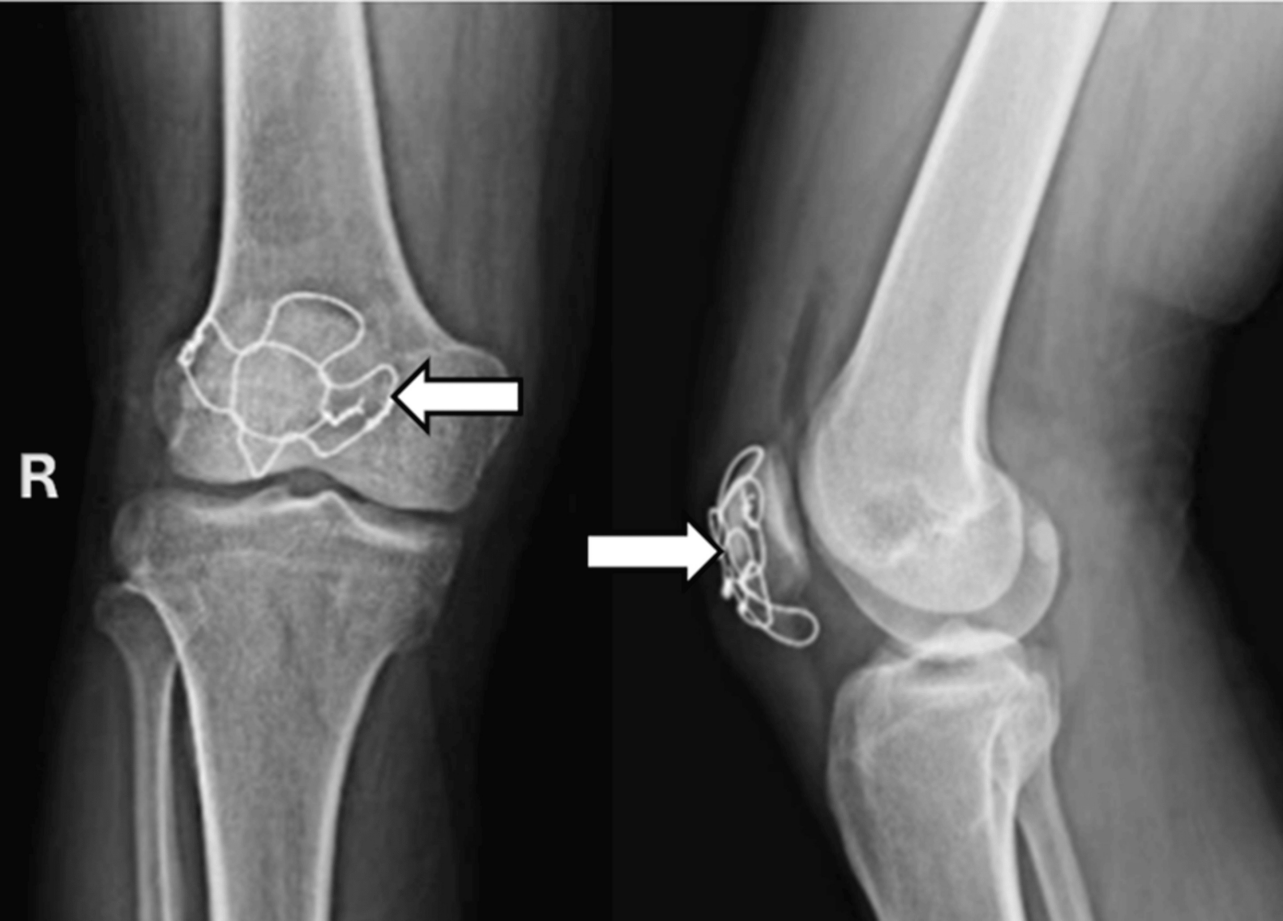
Postoperative radiograph showing patella fixation with the modified triple fixation technique
